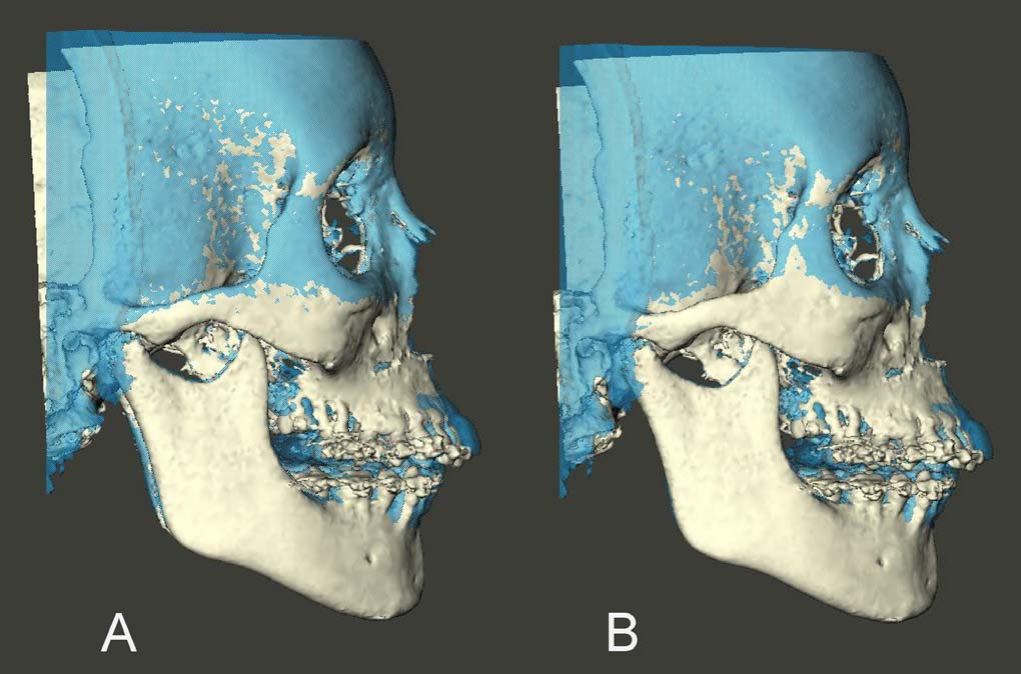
Transparency overlay of superimposed 3D CBCT models. Right side view. (A) models registered on the anterior cranial base. (B) same models registered on the left zygomatic arch.
